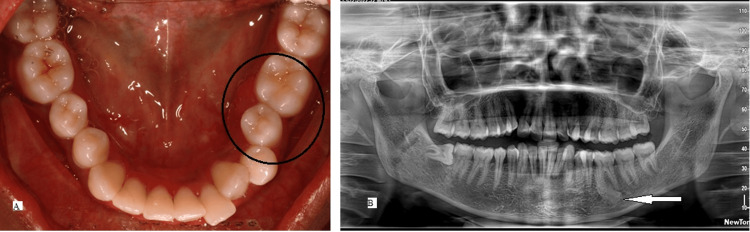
A 21-year-old female presented with malocclusion (A) Intra-oral mandibular arch view shows an expansile lesion lingual to 35 and 36 (black circle) with no remarkable changes in the overlying mucosa. (B) OPG reveals a solitary, unilocular, well-defined radio-dense lesion measuring 7 × 15 mm, located in the posterior mandible and centered at the mesiobuccal root apex of 36 (arrow). OPG, orthopantomograph
